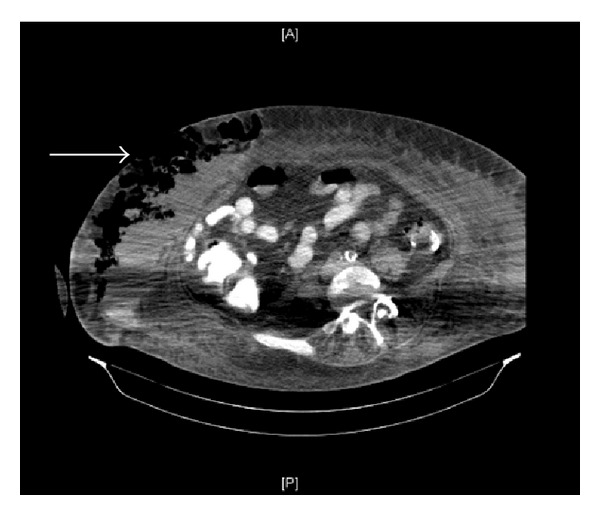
CT image of the abdomen. The arrow indicates extensive subcutaneous collection of fluid and air in the abdominal wall.
